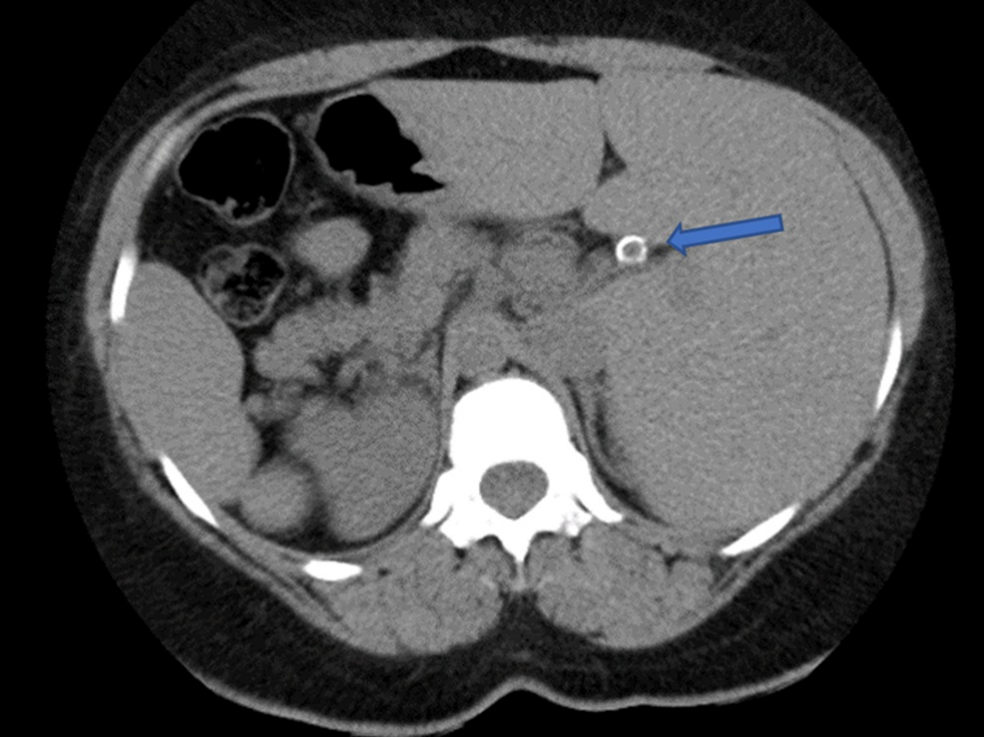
Plain CT of abdomen (axial view) showing right-sided spleen, left-sided liver with incidental finding of gallstones (Blue arrow). CT - computed tomography
